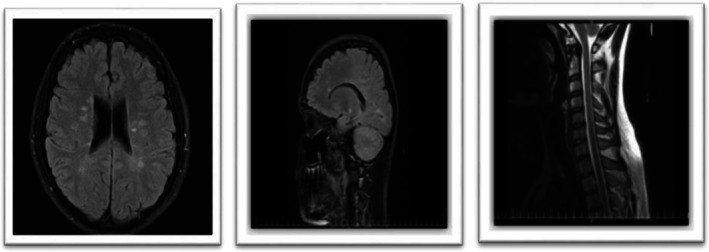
Baseline MRI at fingolimod initiation. Axial FLAIR brain MRI shows stable, non‐enhancing periventricular white matter lesions, consistent with the patient's known multiple sclerosis. And sagittal T2‐weighted cervical spine MRI at the same time demonstrates no evidence of myelitis or active spinal cord lesions.
